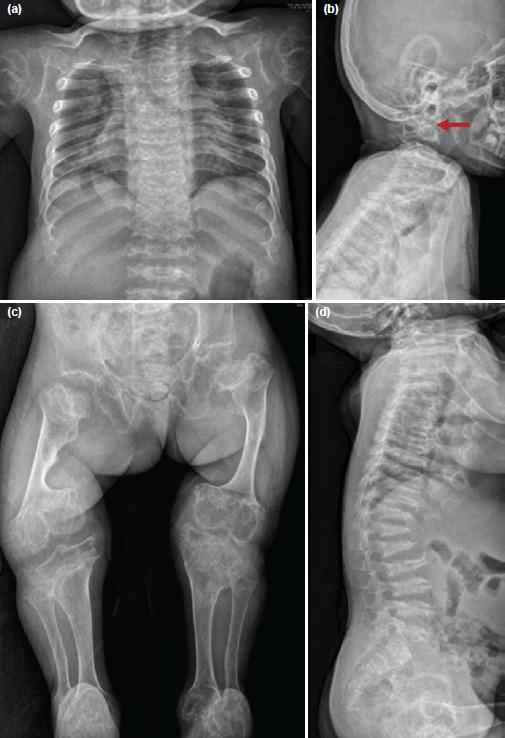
(a) Chest Radiograph shows narrow thorax with broad oar shaped ribs. (b) Lateral cervical spine shows severe odontoid hypoplasia. (c) Halberd Pelvis with short wide ilia, narrow sciatic notches, dysplastic acetabuli, coxa vara and breva, short and thick diaphysis with dumbbell shaped ends, prematurely fused physis, flexion deformity at the knees, fibulae are shorter than tibiae. (d) Lateral spine radiograph shows thoracic lumbar kyphosis, platyspondyly with anterior wedging and characteristic axial split of the lumbar vertebrae body.
